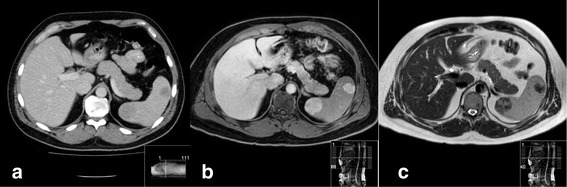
a Computed tomography scan of the abdomen showing two hypodense lesions of the spleen. b, c Magnetic resonance imaging of the abdomen showing the two lesions in T1 and T2 mode, respectively
